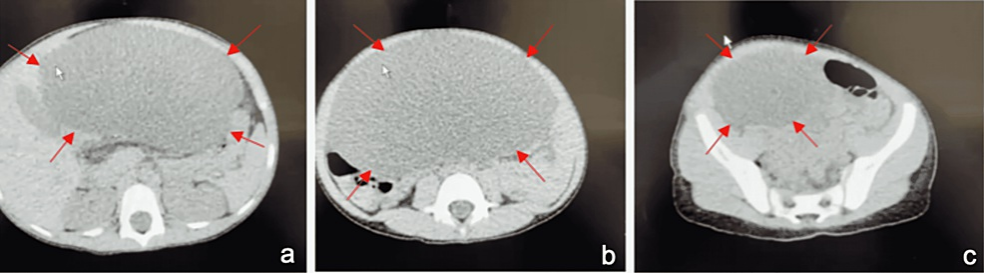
Non-contrast CT of the abdomen. Images a, b, and c show different sections of the cyst. Red arrows mark mesenteric cyst.
